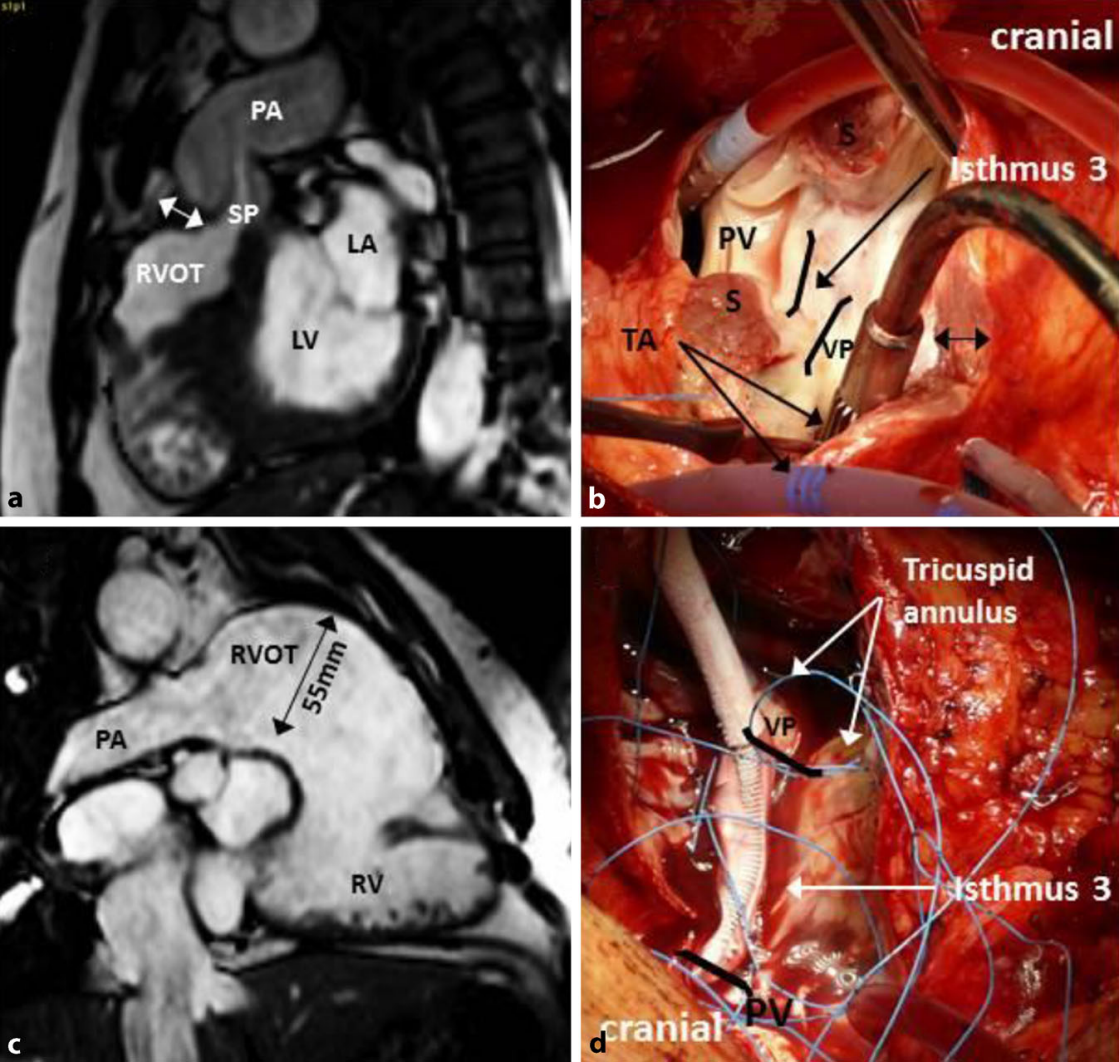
Cardiac magnetic resonance imaging (MRI; a) and intraoperative findings (b) of a patient with repaired tetralogy of Fallot (rTOF) and residual subpulmonary stenosis without ventricular tachycardia (VT). Isthmus 3 bordered by the ventricular septal defect (VSD) patch and the PV consisting of fibrous tissue precluding its participation in VT. c Cardiac MRI of a patient with rTOF and severe PV regurgitation with RVOT dilatation and VT related to a slow-conducting anatomical isthmus 3. Intraoperative cryoablation of isthmus 3 connecting the PV and the VSD patch (d). *PA* pulmonary artery, *SP* subpulmonary stenosis, *RVOT* right ventricular outflow tract, *LA* left atrium, *LV* left ventricle, *PV* pulmonary valve, *TA* tricuspid annulus, *VP* VSD patch
